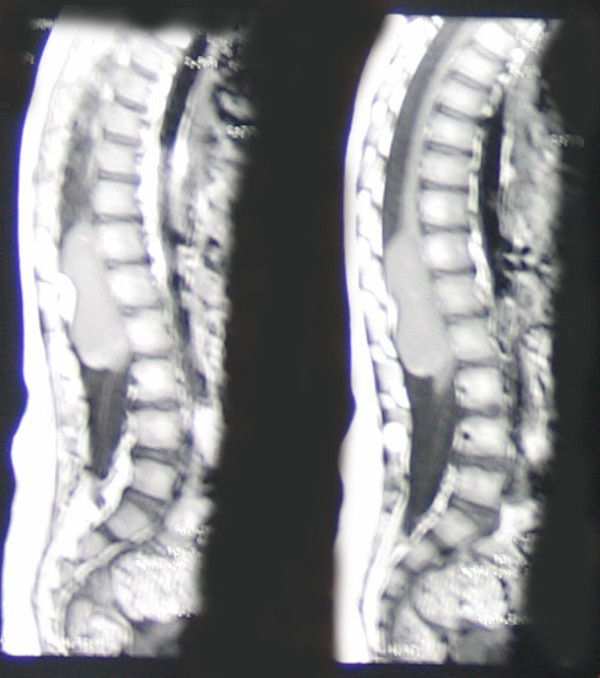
Sagittal T1-weighted MRI scan showing an isointense tumor with fat signal on the dorsal surface.
